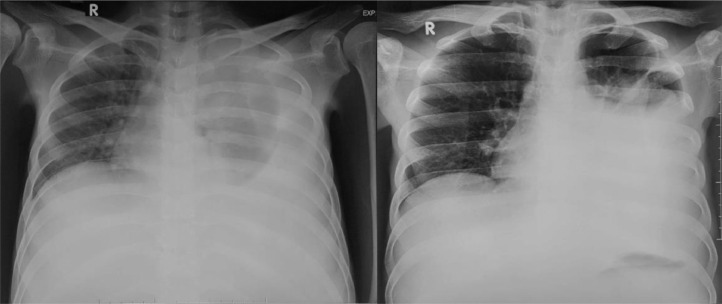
Plain chest radiograph (A) on December 2021 and (B) on January 2022, in which the effusion significantly increased.
